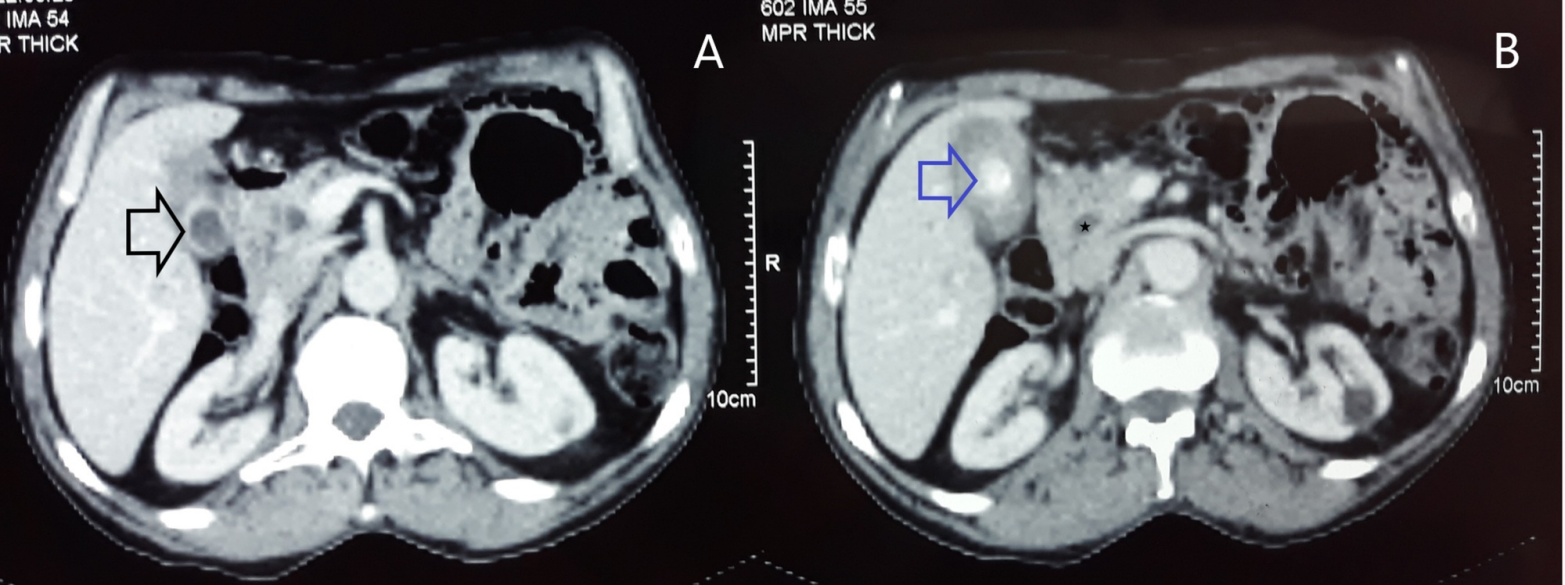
(A and B) Computed tomography scan showing irregular thickening of the gallbladder wall (black arrow) with the presence of a large calculus in the gallbladder (blue arrow), with dilatation of the common bile duct (star).
